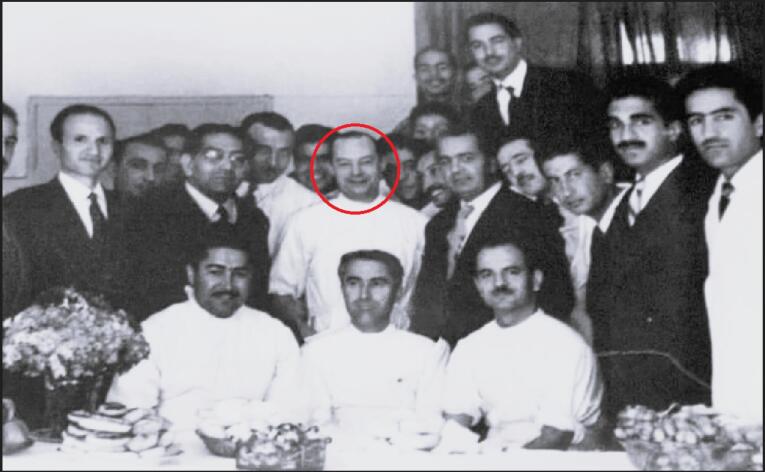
As the head of the department of surgery, he noticed some major defects and tried to correct them. As a result of his efforts, on the morning of August 23, 1964, the modern operating theater of Imam Reza Hospital (including five separate operation rooms) was opened in the presence of local authorities of the province. He reorganized the rooms with modern equipment, such as central X-ray imaging devices. In addition, Dr. Boulvin started renewing the human resources in surgery ward of the hospital. He rearranged the medical staff and nursing teams and implemented regular night shifts for general practitioners and surgery residents. Thus, many European-experienced nurses like Mlle. M. Mary Tayar, who became one of the best colleagues of Dr. Boulvin, were invited to the Department of Surgery. Boulvin also established a blood bank to revolutionize patient management in the hospital.^2^
